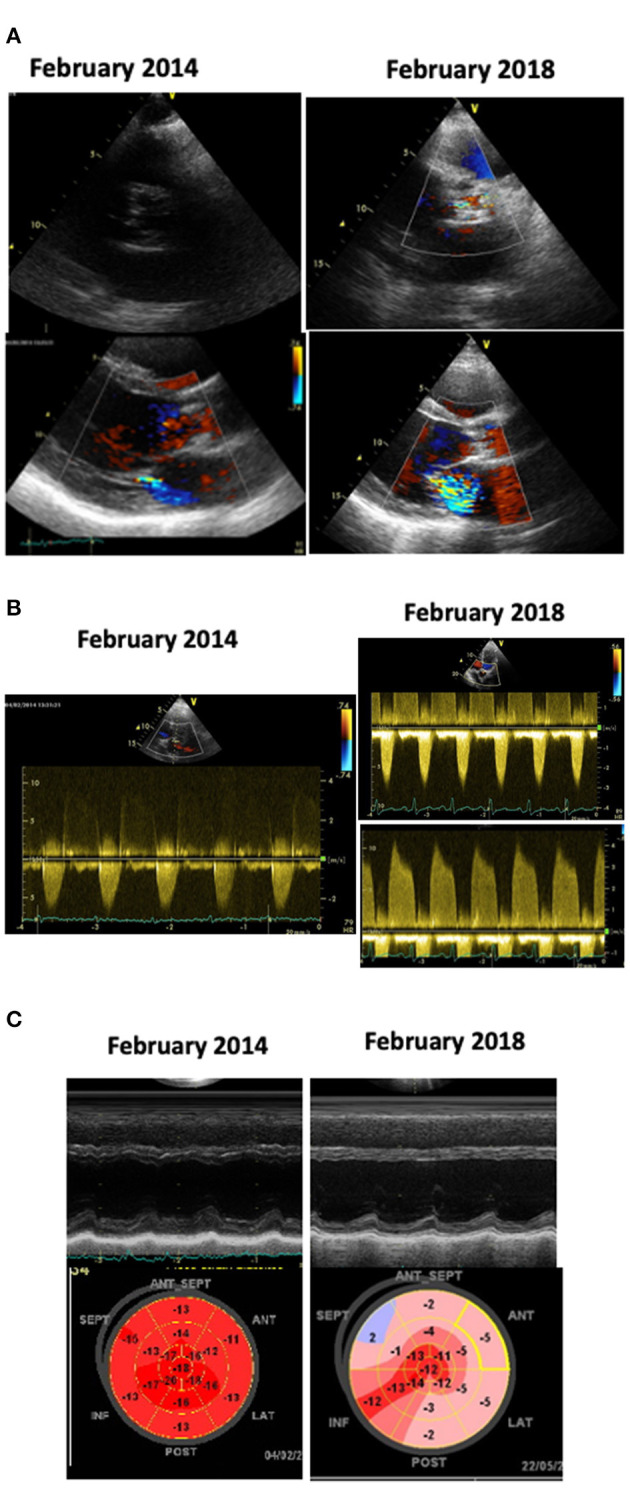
Echocardiograms of a patient treated with anthracyclines chemotherapy and mediastinal RT in 1999, at age 19. He underwent a cardiologic follow-up every 2–3 years. In February, 2014 (images on the left) the echocardiogram detected the new appearance of mild aortic and mitral dysfunction (A,B); the left ventricular (LV) function and Global Longitudinal Strain (GLS) were normal (C); a stress test was negative. We planned yearly check-up, but the patient, who felt completely asymptomatic, skipped the appointments. In February 2018, at age 38, he suddenly experienced a congestive heart failure. The echocardiogram (images on the right) showed: calcific aortic stenosis, severe mitral regurgitation (A); severe aortic stenosis and moderate aortic regurgitation (B); severe LV dysfunction and abnormal GLS (C). At coronary angiography a 70% stenosis of the left anterior descending coronary artery was detected.
